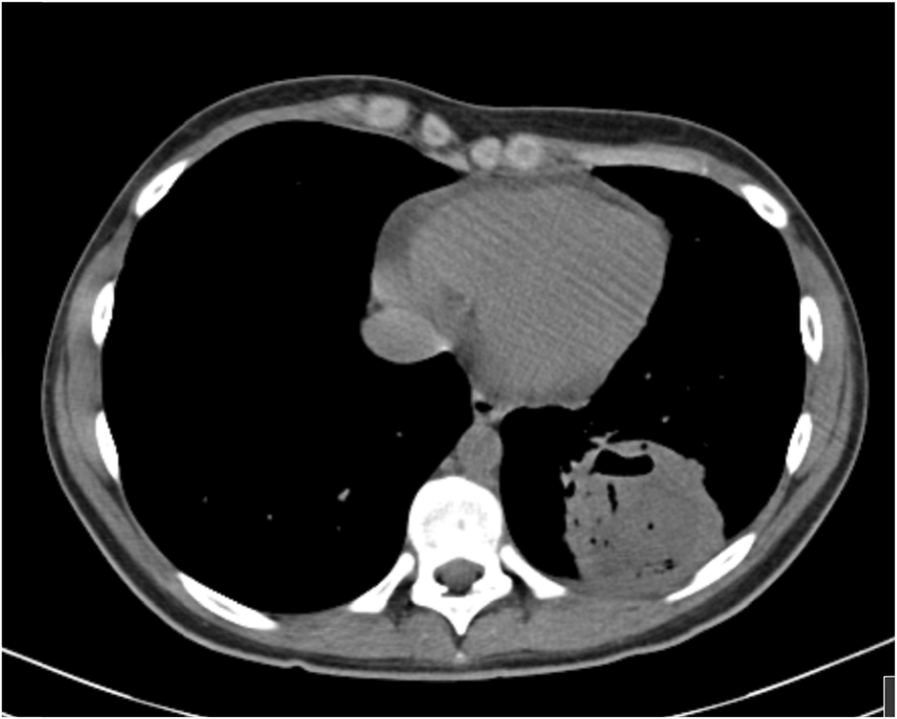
Transverse plane of the chest CT scan showing the presence of the abscess
